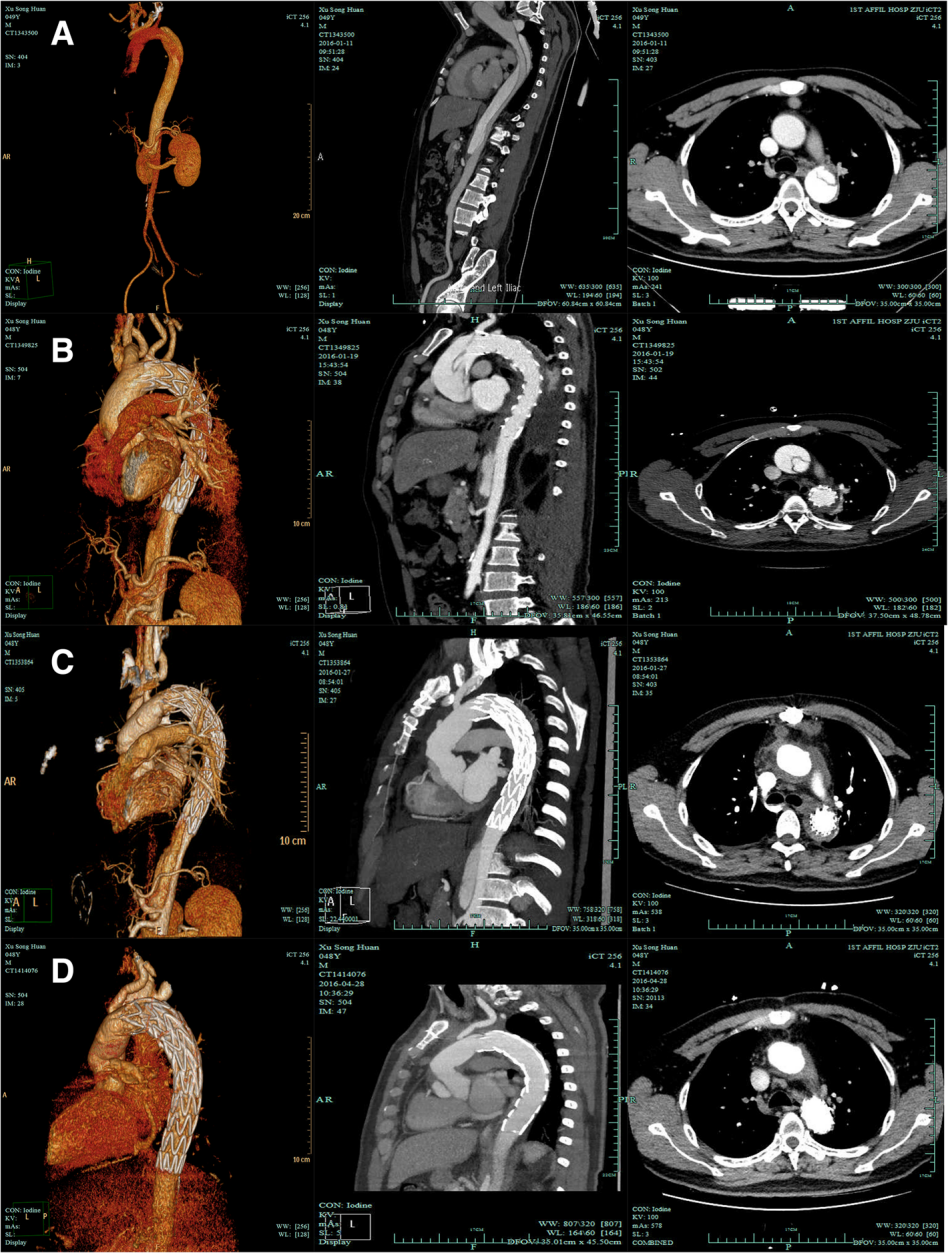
Computed tomography angiography (CTA) of a patient with RTAD related to TEVAR. a preoperatively, CTA showing type B dissection. b CTA reveals dissection of the ascending aorta 8 days after TEVAR. c The true lumen of the ascending aorta returned to normal after surgery. (D) Follow-up of CTA at 3 months, the false lumen thrombosis
